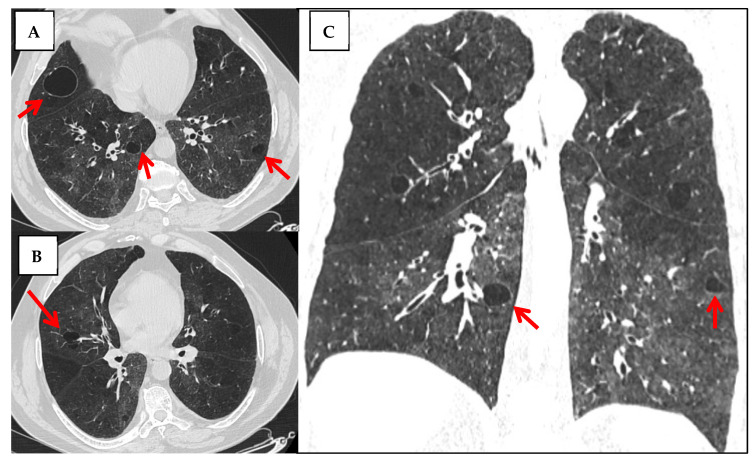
HRCT Features Suggestive of Lymphocytic Interstitial Pneumonia in a Patient with Suspected Autoimmune Disease. Axial (A,B) and coronal (C) views show multiple thin-walled cysts with peribronchovascular and subpleural distribution, predominantly in the lower lobes (red arrows). Patchy areas of ground-glass opacity are seen throughout both lungs, along with ill-defined centrilobular and subpleural nodules. Subtle peribronchovascular interstitial thickening is also noted. This constellation of findings—diffuse ground-glass opacities, cystic change, nodularity, and perilymphatic distribution—is highly suggestive of LIP, particularly in the clinical context of autoimmune disease such as Sjögren’s syndrome.
